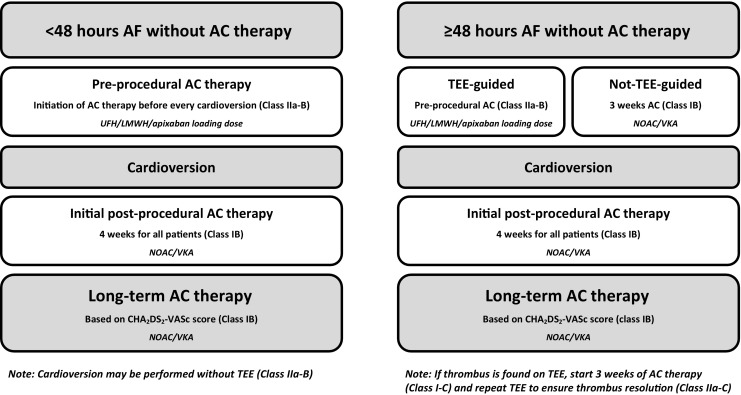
Pericardioversion anticoagulation: key recommendations in the ESC 2016 AF guidelines (*ESC* European Society of Cardiology, *AF* atrial fibrillation, *AC* anticoagulation, *UFH* unfractionated heparin, *LMWH* low-molecular-weight heparin, *NOAC* non-vitamin K oral anticoagulant, *VKA* vitamin K antagonist, *CHA*_*2*_*DS*_*2*_*-VASc score* Congestive heart failure, Hypertension, Age ≥ 75 [doubled], Diabetes, prior Stroke [doubled]—Vascular disease, Age 65–74, Sex category, *TEE* transoesophageal echocardiography)
